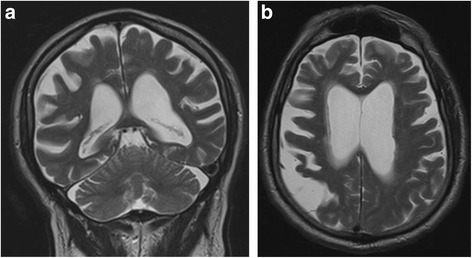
Atrophy and extensive gliosis of the left frontoparietal region in severe Alzheimer’s disease. Images (a) and (b) depict volume loss in end-stage Alzheimer’s disease with mild changes to the periventricular white matter. a Coronal T1-weighted magnetic resonance imaging scan showing marked progressive cortical atrophy of the parietal regions. b Transverse T1-weighted magnetic resonance imaging scan showing bilateral marked atrophy
